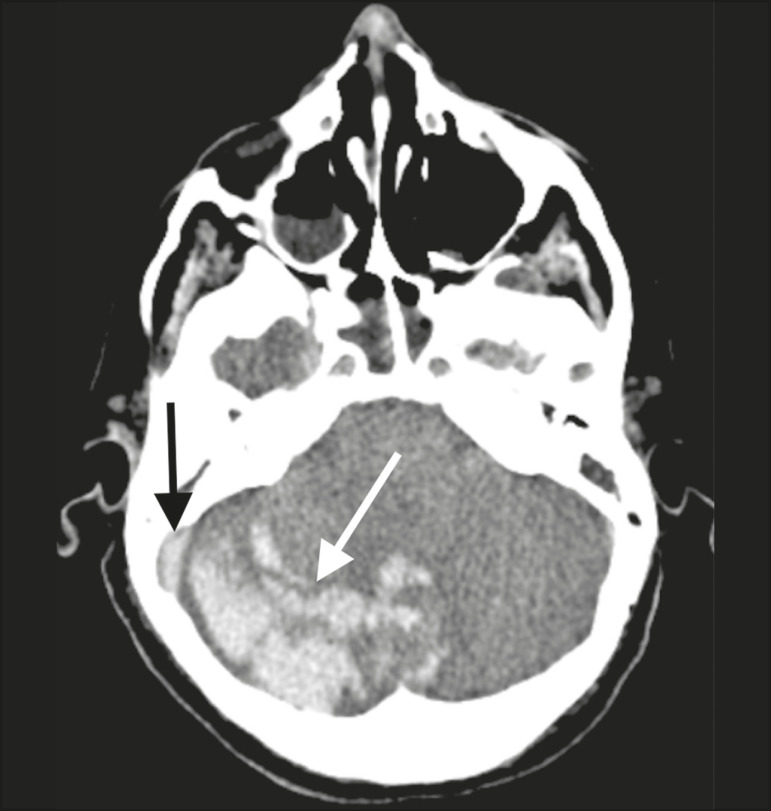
Case 4. Brain CT of a 56-year-old woman with COVID-19 showing a hematoma in the vermis and right cerebellar hemisphere (white arrow), together with a hyperdense area throughout the ipsilateral sigmoid and transverse sinuses (black arrow) suggestive of dural sinus thrombosis as the cause of the cerebellar hematoma.
